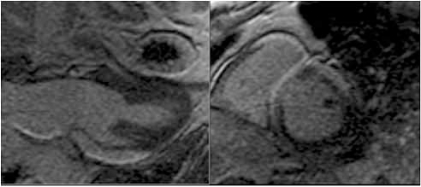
Cardiac MRI Showing Sarcoid Infiltration.
